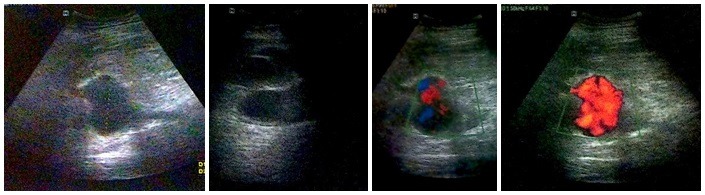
Ultrasonographic appearance of the femoral artery aneurysm.
